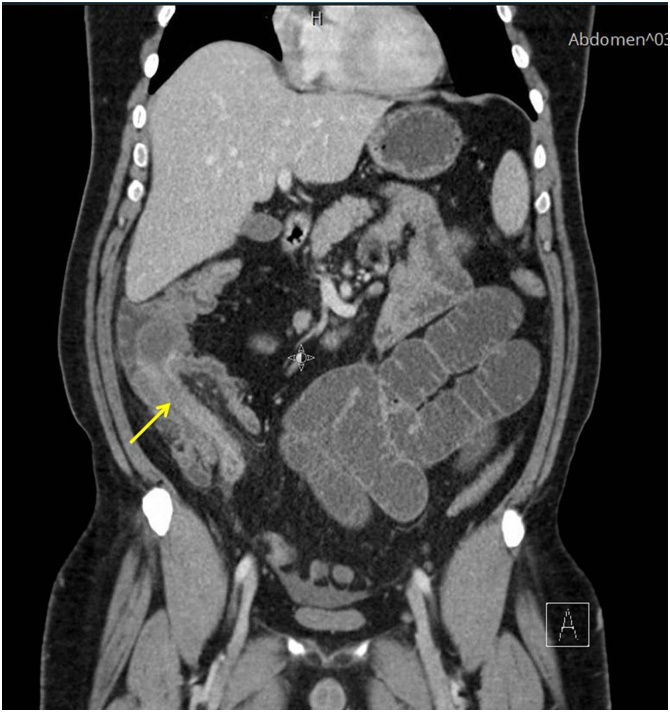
Coronal CT Scan with intravenous contrast demonstrating the intussusception of the small bowel into the ascending colon with the characteristic of double configuration of the intestinal wall (yellow arrow).
